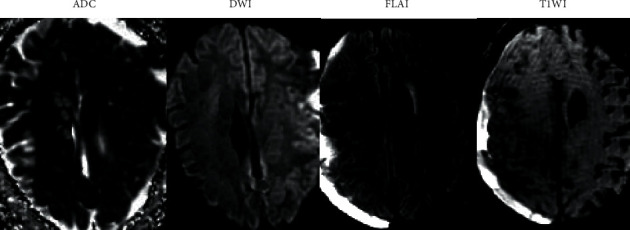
MRI images of early ischemic stroke in the subacute phase.
